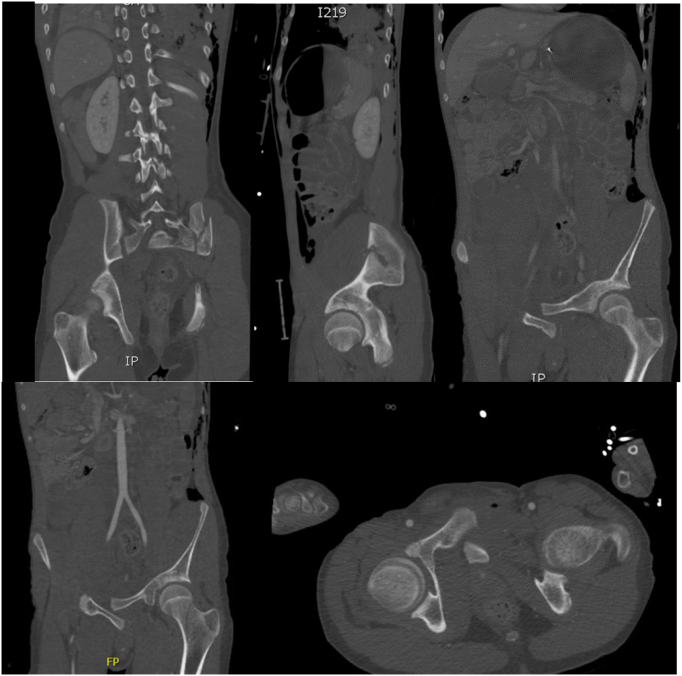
CT scan of the pelvis shows displaced fracture of the left iliac bone, commuted left sacral ala fracture, minimally displaced right-sided sacral ala fracture and complete rupture of the pubic symphysis with LSP.
